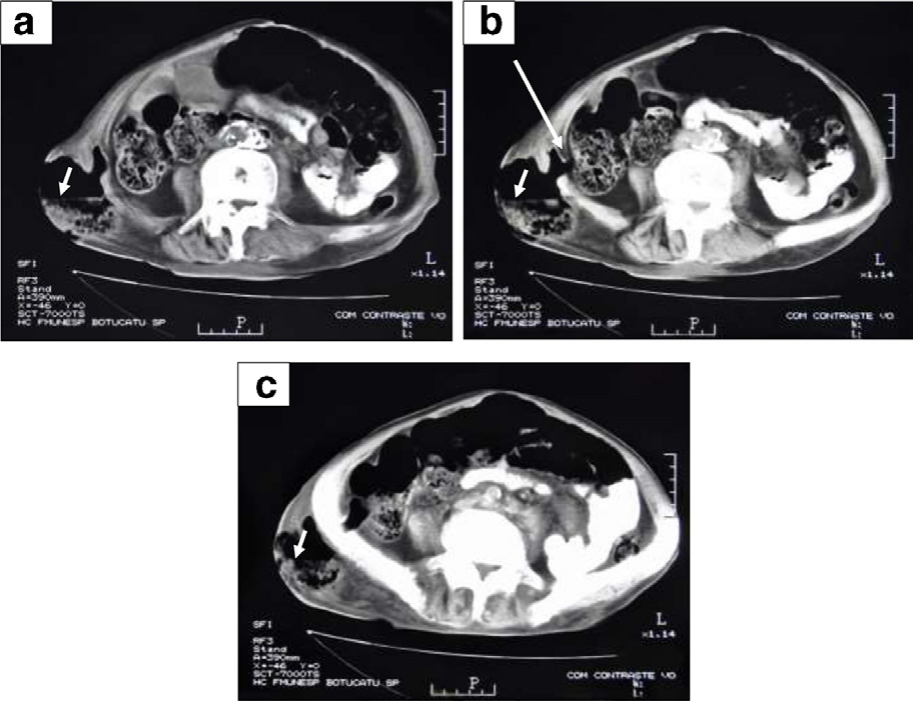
﻿Figure 1- a, b, c: CT scan with oral contrast: axial sections showing a faecal fistula crossing through the right iliac bone in its way from the colon to a gluteous reservoir. Complete fistula track can be seen only on Figure 1b (big arrow). Other pictures correspond to images taken respectively above (Figure 1a) and below (Figure 1c) that one. An air-feces level can be seen in the gluteous reservoir (small arrows).
